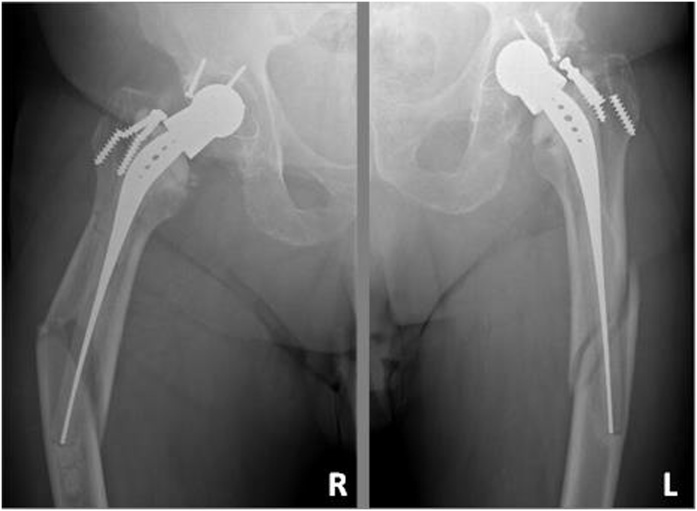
Preoperative anteroposterior radiographs of the hips showing bilateral isoelastic prosthesis with osteolysis along the whole length of the femoral stem after 24 and 21 years in situ. Broken screws due to the prosthetic instability.
